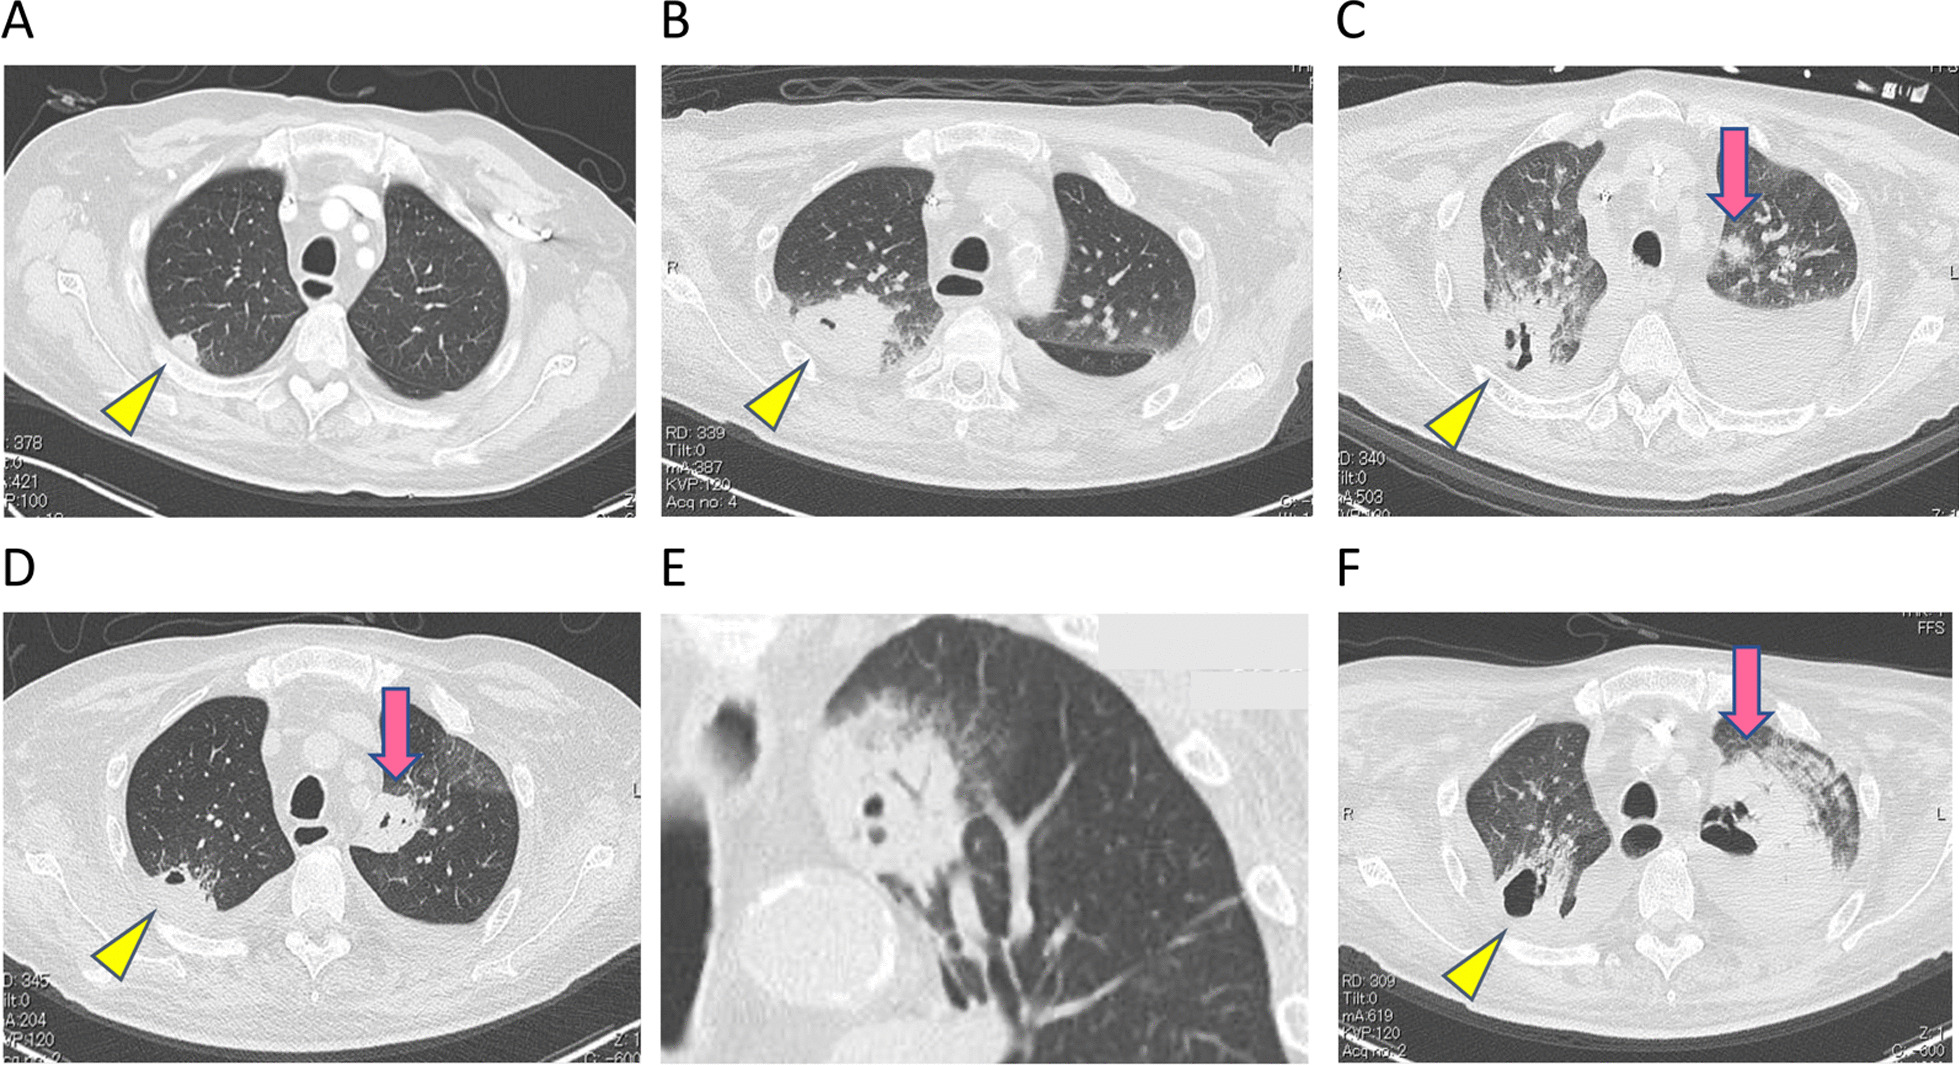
The transition of the patient’s CT imaging. A–D, F Axial CT images on day 28 (A), day 41 (B), day 53 (C), day 61 (D), and day 71 (F). A A nodule in the peripheral region of the right upper lobe (arrowhead). B Enlargement of the nodule with newly emerged cavitation in the right upper lobe (arrowhead). C A new nodule emerges in the left upper lobe (arrow). D The nodule in the left upper lobe is enlarged with cavitation (arrow), whereas the nodule in the right upper lobe is diminished (arrowhead). E A coronal CT image on day 61. The nodular lesion in the left upper lobe shows cavitation with the halo sign. F Rapid growth of the nodule in the left upper lobe (arrow)
